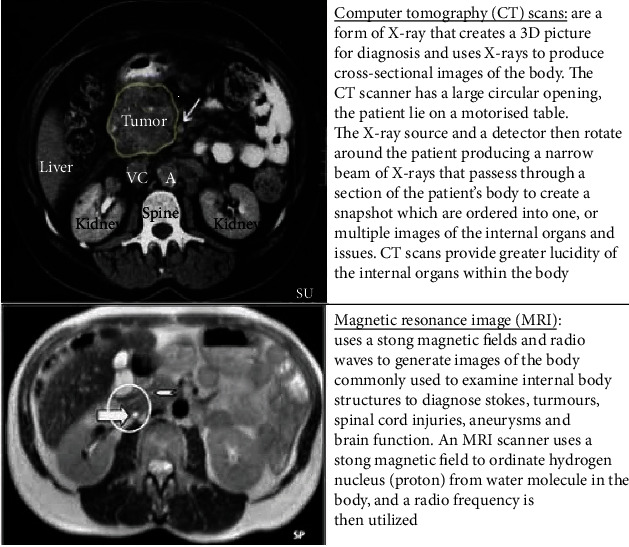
Medical imaging techniques [9–12]: images from CT and MRI modalities.
